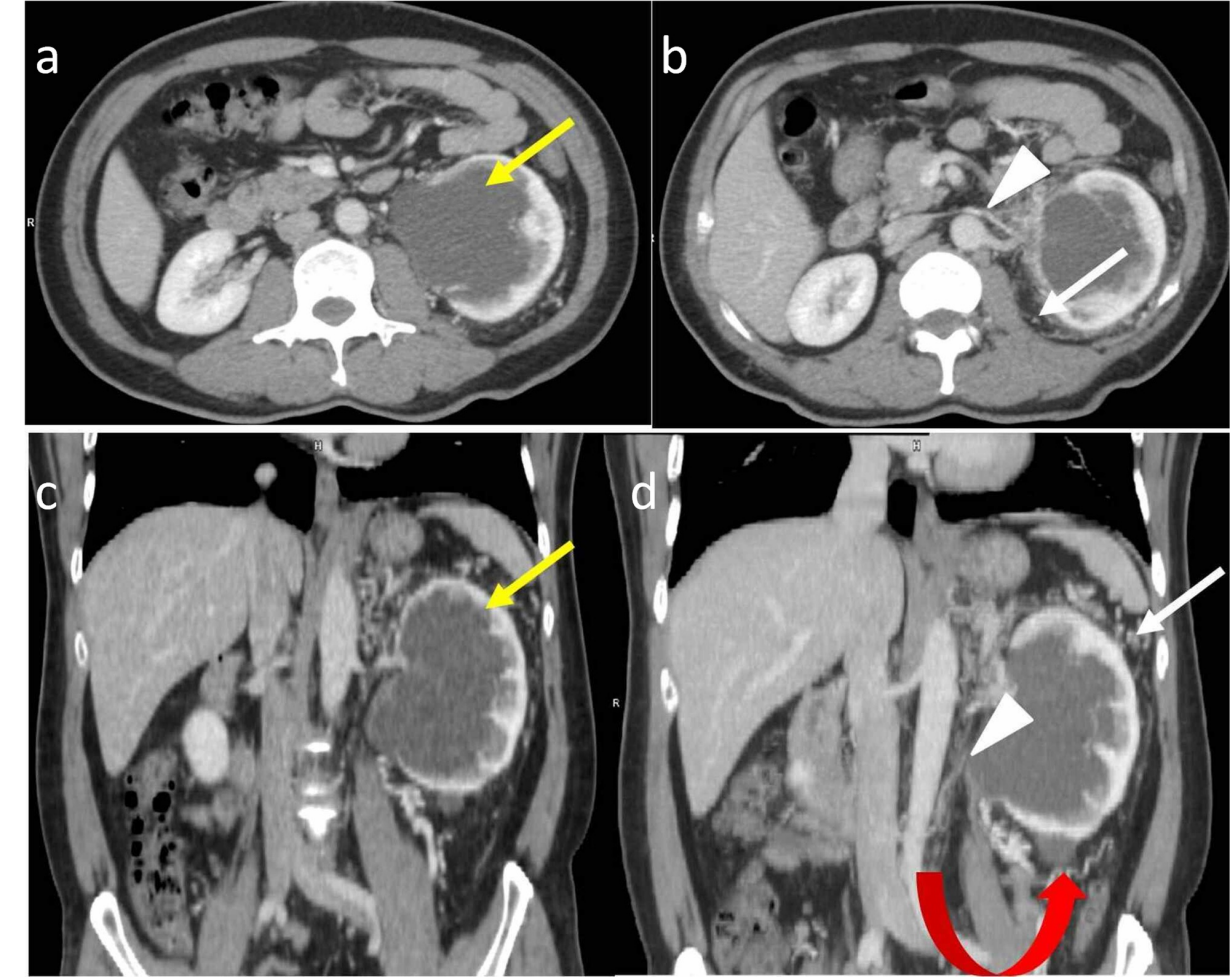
49-year-old male with left peripelvic renal lymphangiectasia Contrast-enhanced CT of the abdomen in the venous phase in axial (a, b) and coronal planes (c, d) demonstrates a peripelvic cystic lesion (yellow arrows) on the left side. It also demonstrates the attenuated caliber of the left renal vein (white arrowheads) with perirenal collaterals (white arrows) consistent with chronic left renal vein thrombosis. Associated Bosniak type I cyst (red curved arrow) is seen in the lower pole of the left kidney CT: computed tomography
